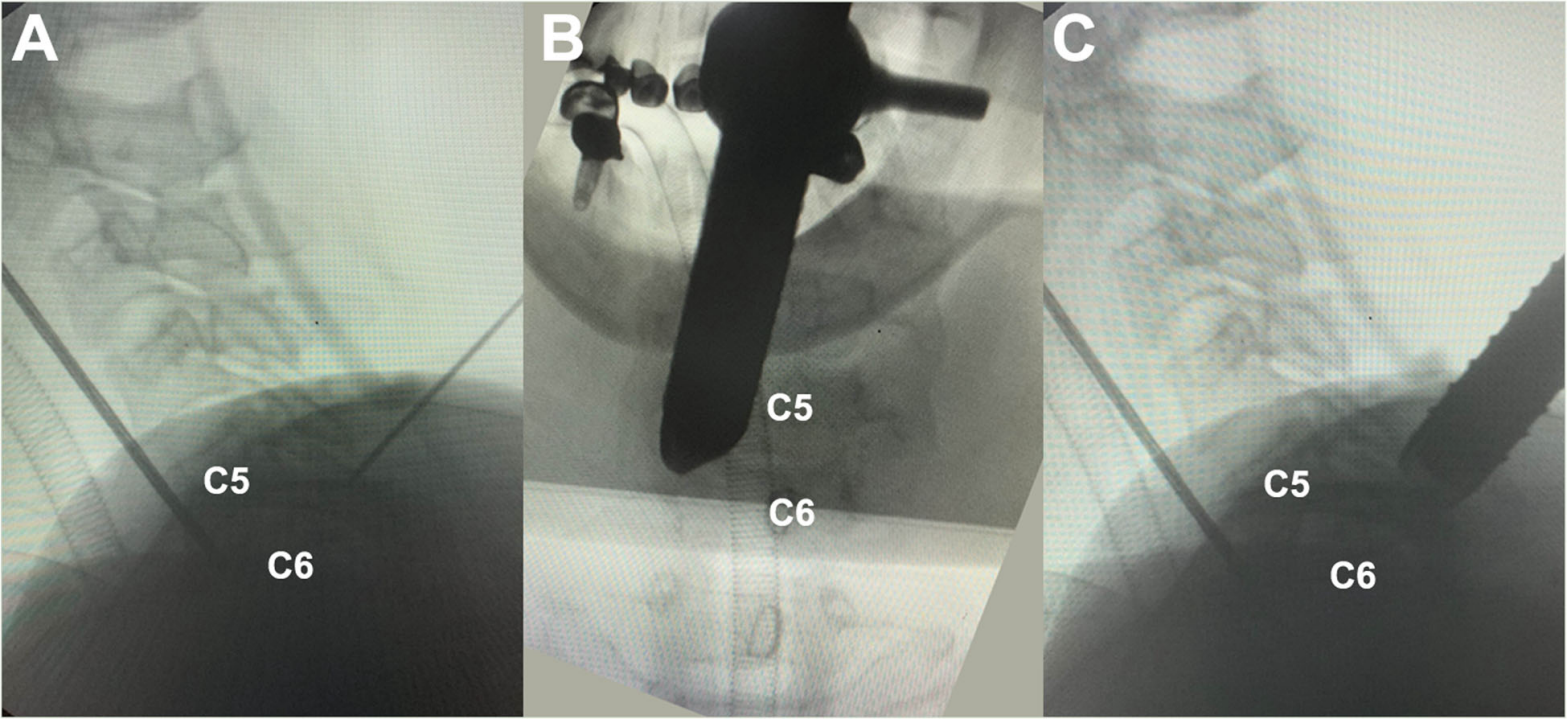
Intraoperative fluoroscopic images during p-PECD with 6.9 mm endoscope. a The C5-C6 level was localized on lateral view; b-c the working cannula is located over the “V” point on radiographs
